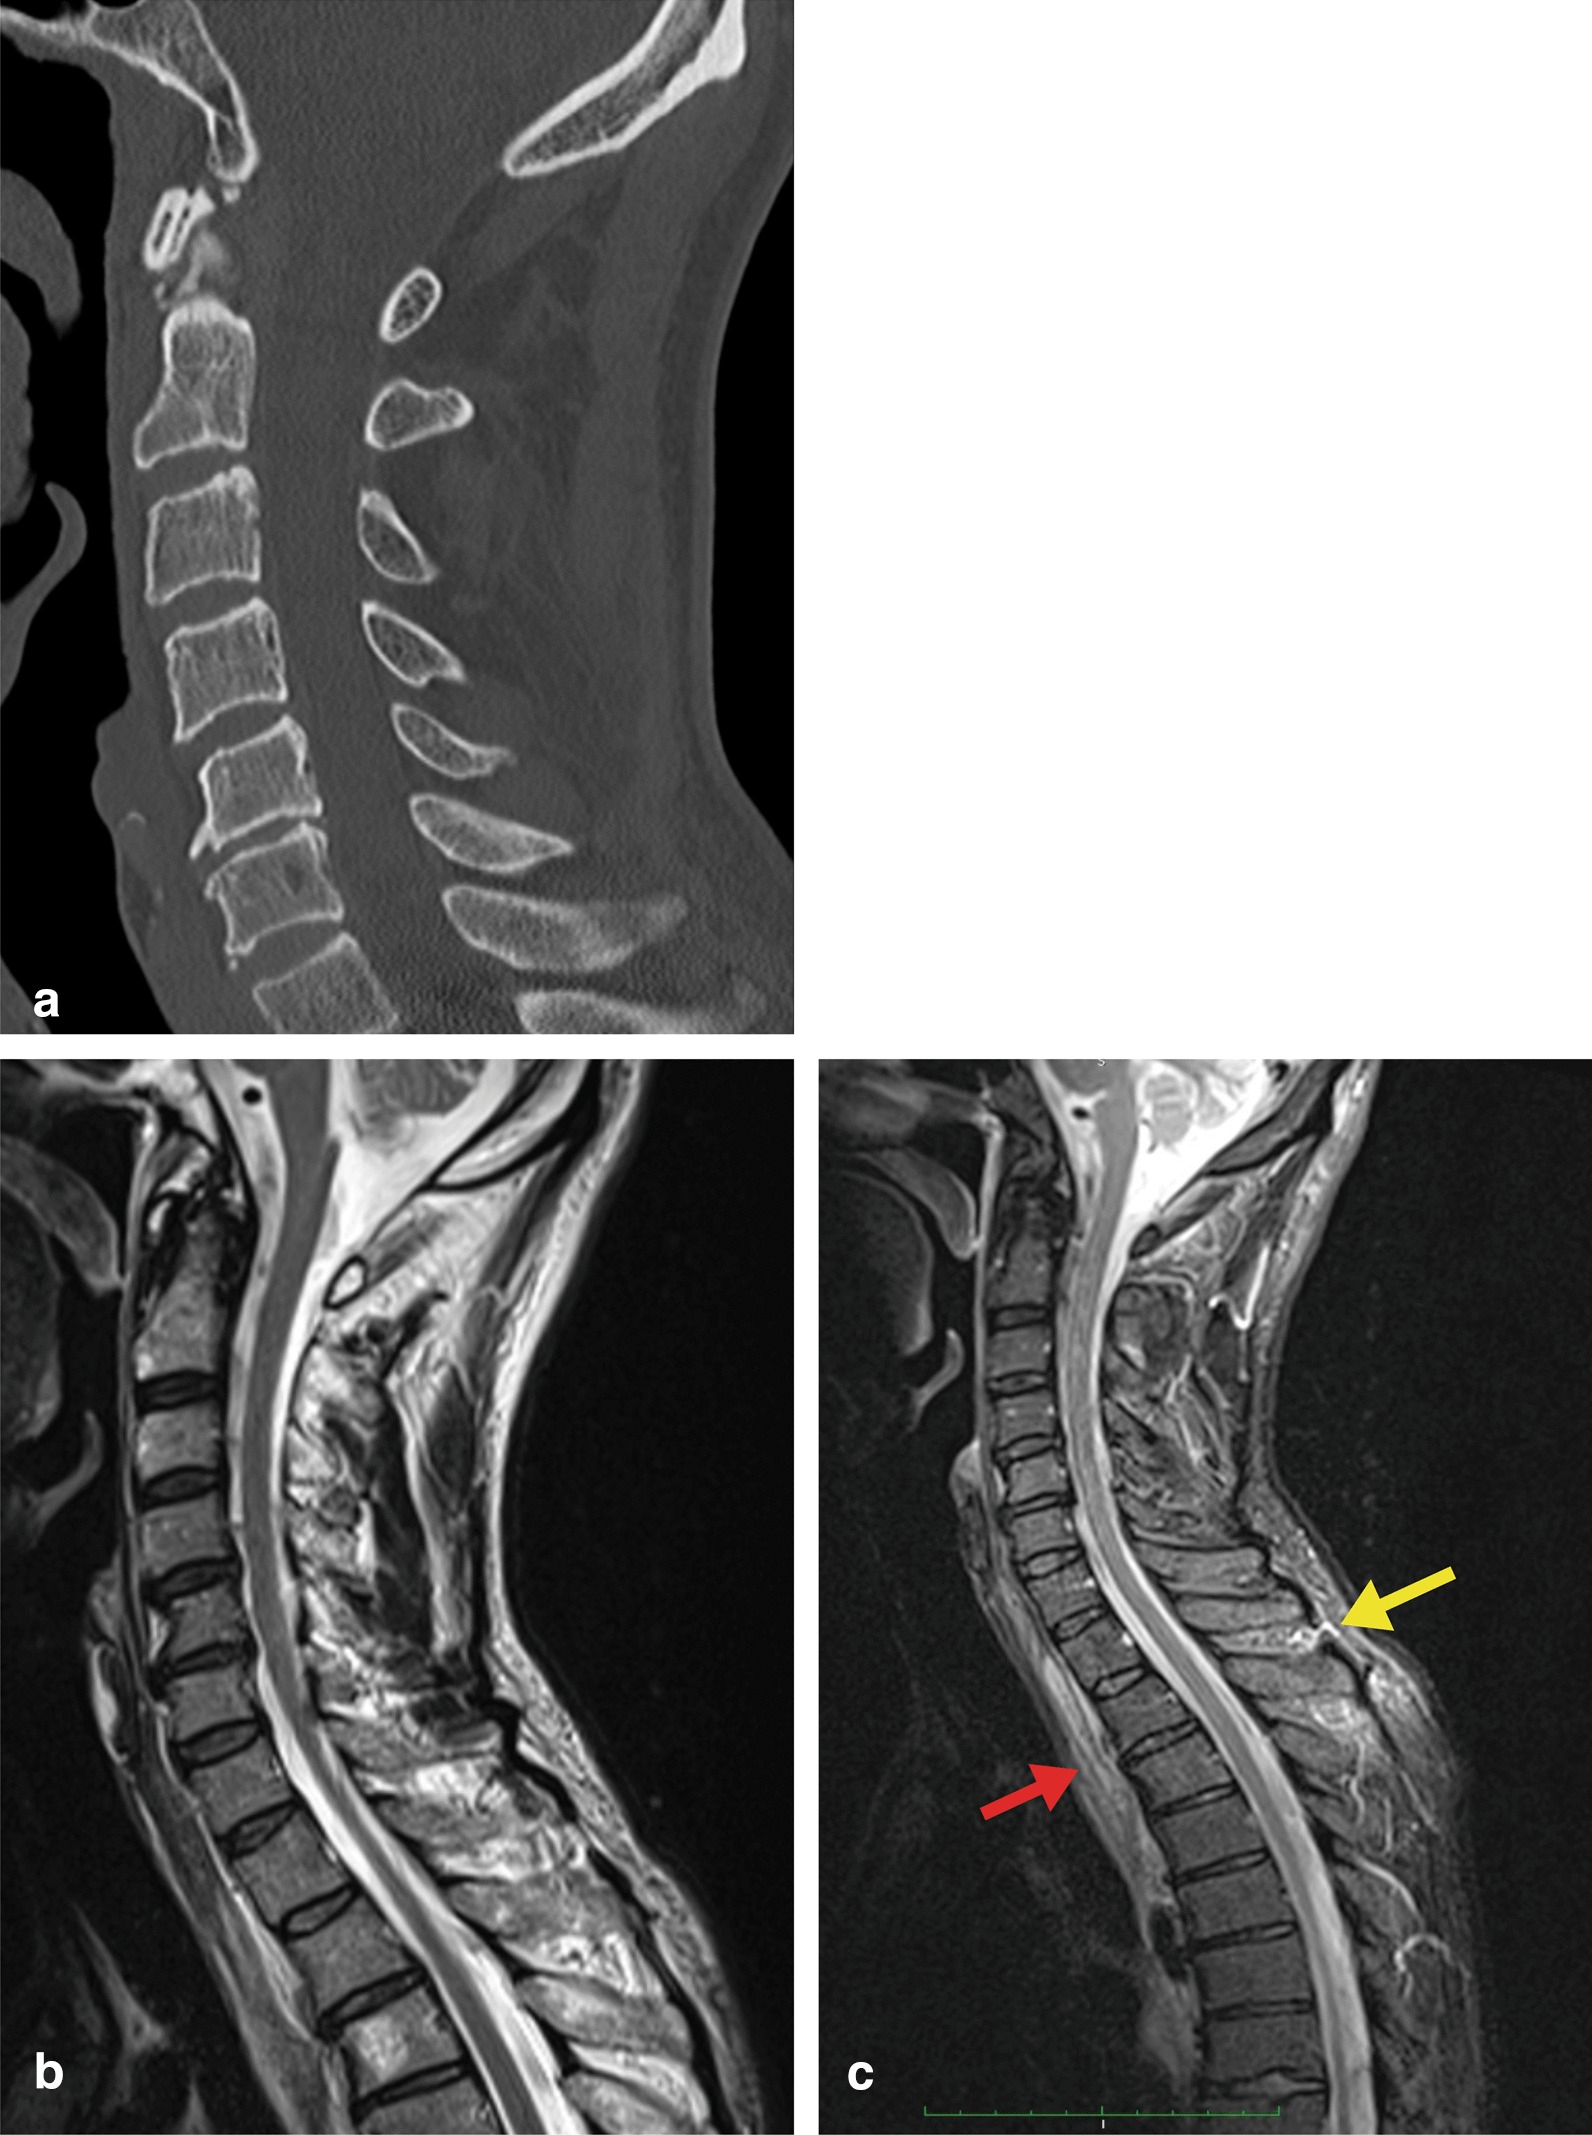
a Computed tomography of the cervical spine on admission demonstrates degenerative changes at C5/6. b T2-weighted magnetic resonance imaging (1.5 T, 3 mm slice thickness) 3 days later was performed because of neck pain without radicular or medullary symptoms. Degenerative changes were confirmed at C4–7, with mild canal stenosis at C4/5 (10.9 mm) and C5/6 (10.2 mm). A hemangioma at Th3 was observed. Myelomalacia was ruled out. c Short-T1 inversion recovery (STIR) magnetic resonance imaging with a large field of view demonstrates subtle prevertebral hemorrhagic effusion within the soft tissue (red arrow) as well as edema between the processi spinosi (yellow arrow) at the cervicothoracic transition
